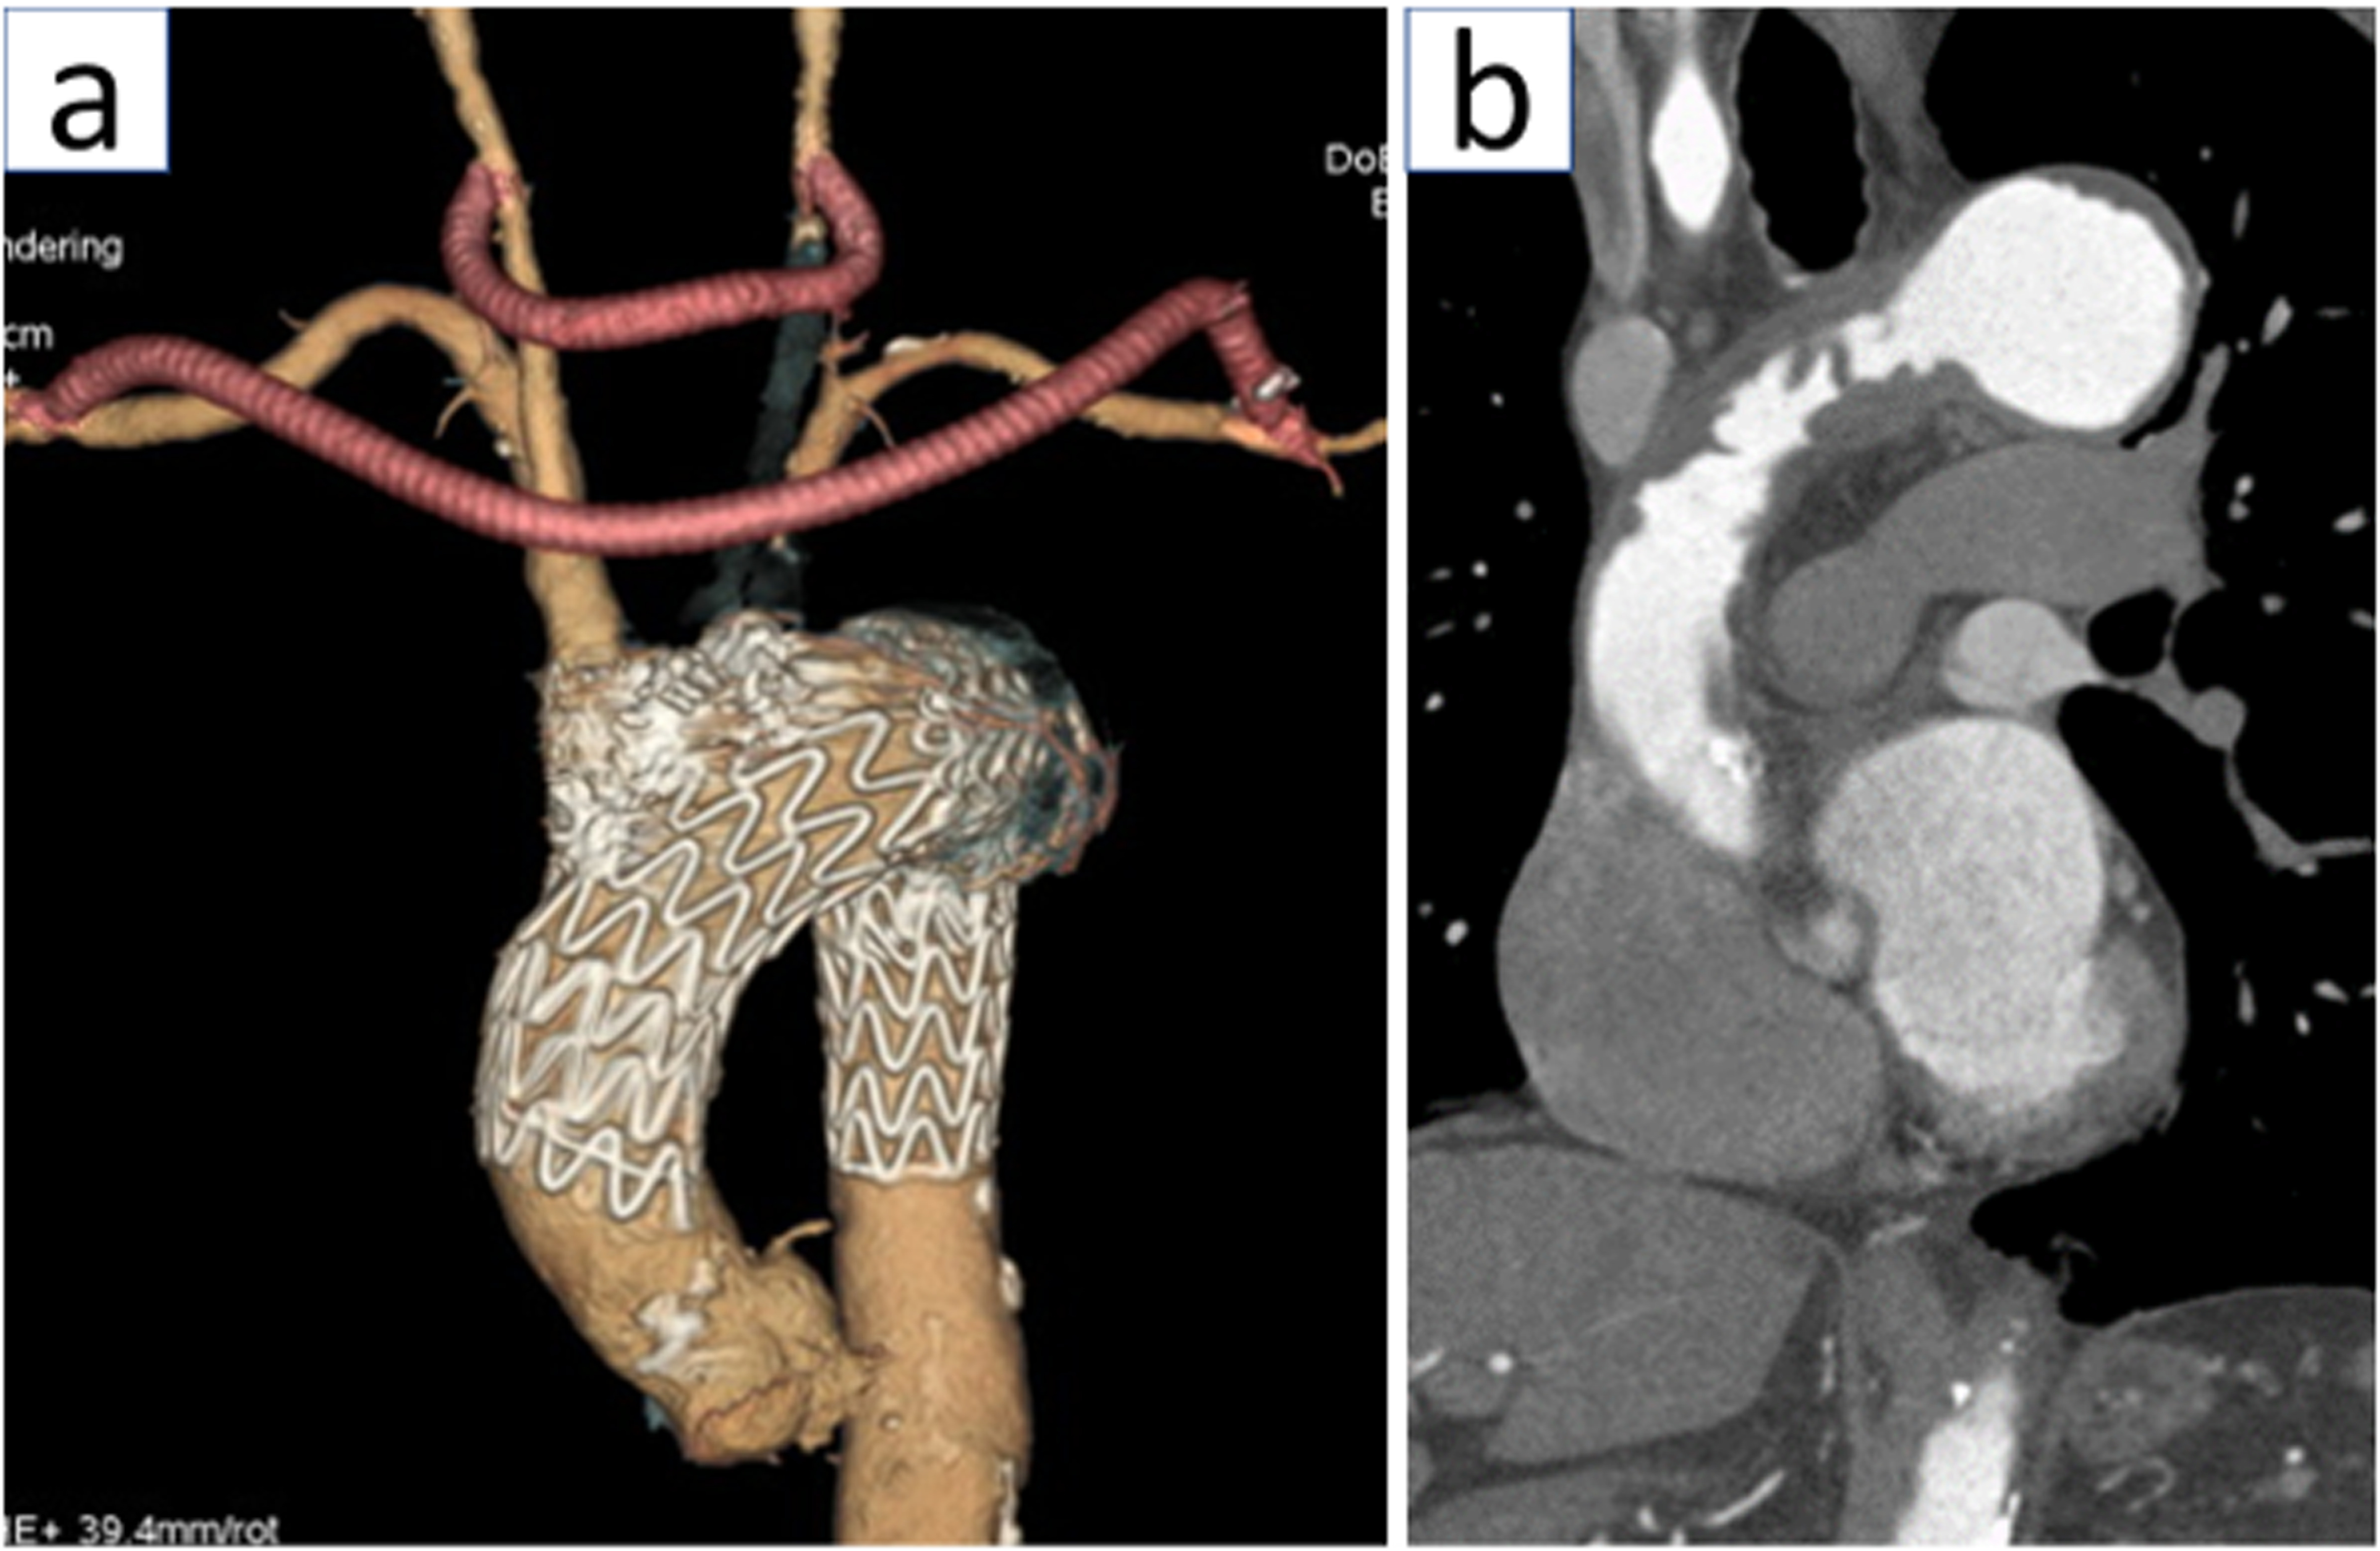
Fig. 2 Image of a practical case that underwent chimney thoracic endovascular aortic repair (TEVAR). (a) Three-dimensional image of completed chimney TEVAR with carotid–carotid bypass and axillo-axillary bypass. (b) Preoperative computed tomography sagital view. Grade IV atheroma was observed in the ascending aorta and aortic arch.
